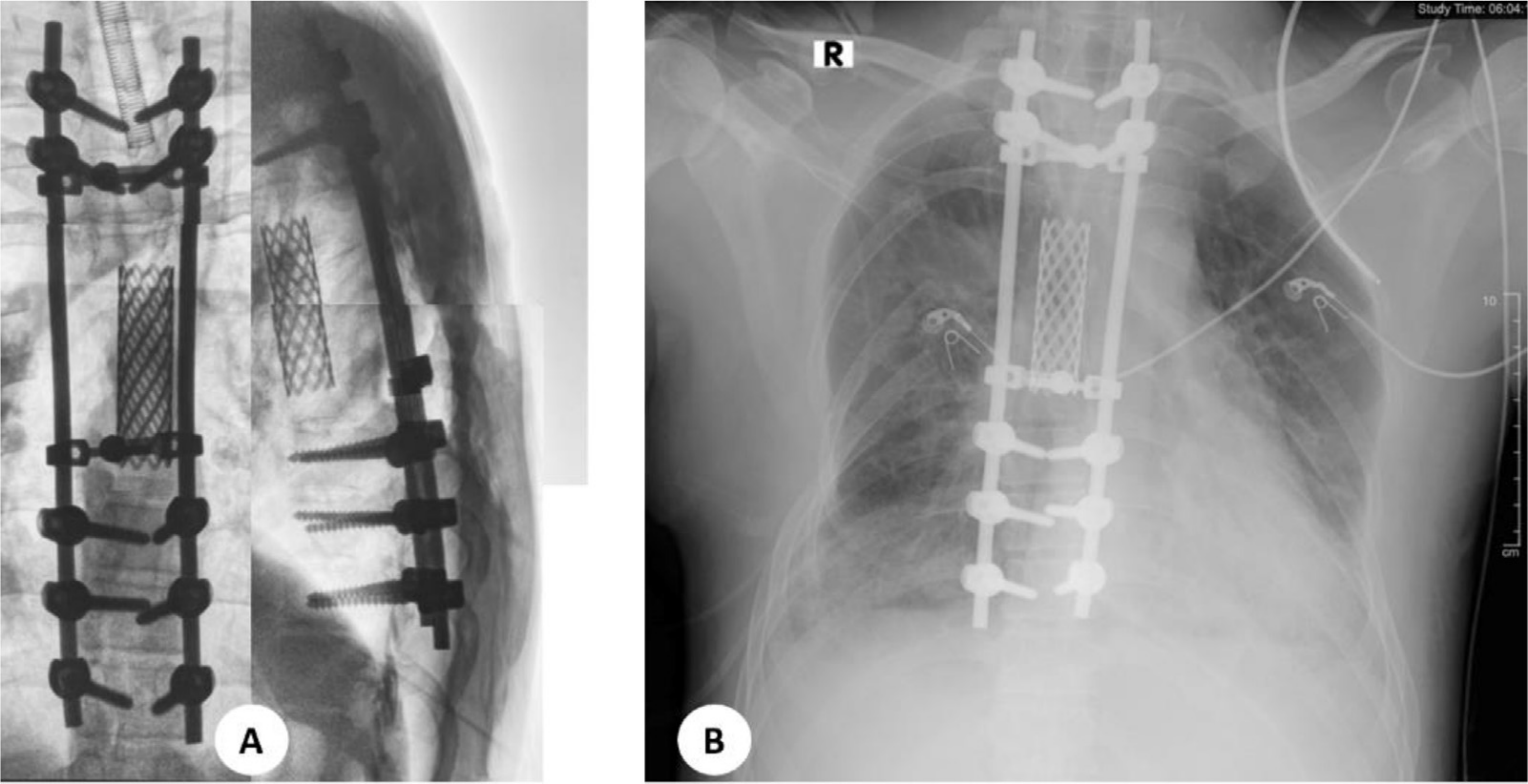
Postsurgery (A) Thoracolumbal X-ray imaging, the T5-T6 thoracic vertebrae bodies are not visualized due to corpectomy. (B) Thorax X-ray imaging, showed no complication from surgery.
